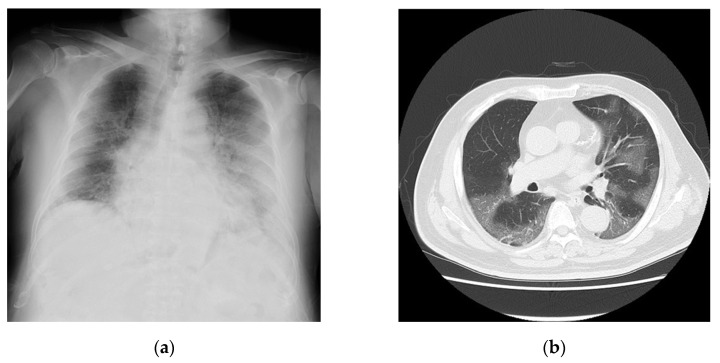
Imaging findings of Case 2 captured on day of admission. (a) Chest radiograph and (b) chest computed tomography image showing bilateral diffuse ground-glass opacities.
